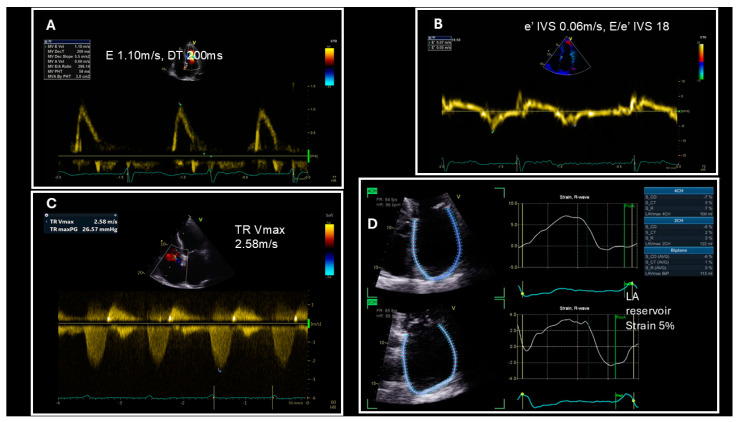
Echocardiographic assessment of left ventricular diastolic dysfunction in an obese patient with atrial fibrillation. (A) E wave velocity is >1 but deceleration time (DT) is >160 ms. (B) E/e’ ratio at the interventricular septum (IVS) is >11. (C) Tricuspid regurgitation (TR) Vmax is <2.8 m/s. Two parameters are positive and two are negative according to the diastolic dysfunction algorithm. (D) Left atrial (LA) reservoir strain is <16%. Hence, since the patient is obese (body mass index > 30 kg/m^2^), the LV filling pressure is elevated.
